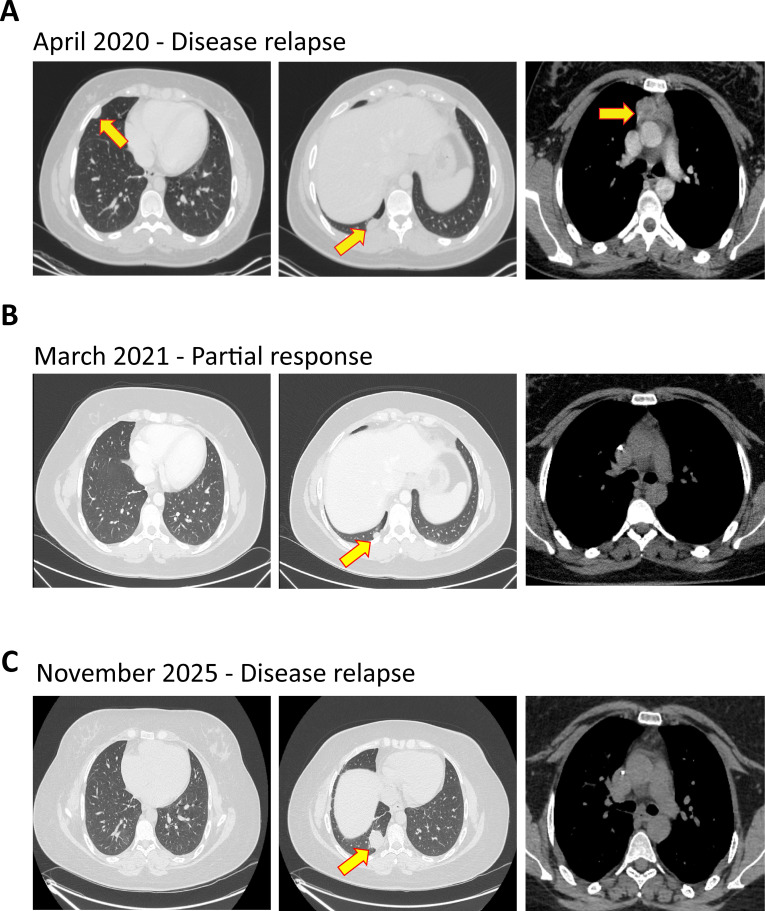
CT scans showing lung localization of the disease (arrows). (A) April 2020: subpleural nodules (left and central panels) and mediastinal adenophaty (right panel). (B) October 2021: residual subpleural nodule (central panel) after immunotherapy, while the previous frontal nodule (left panel), and mediastinal adenopathy (right panel) regressed. (C) November 2025: Pulmonary relapse 21 months after immunotherapy discontinuation. Yellow arrows point the different lesions.
